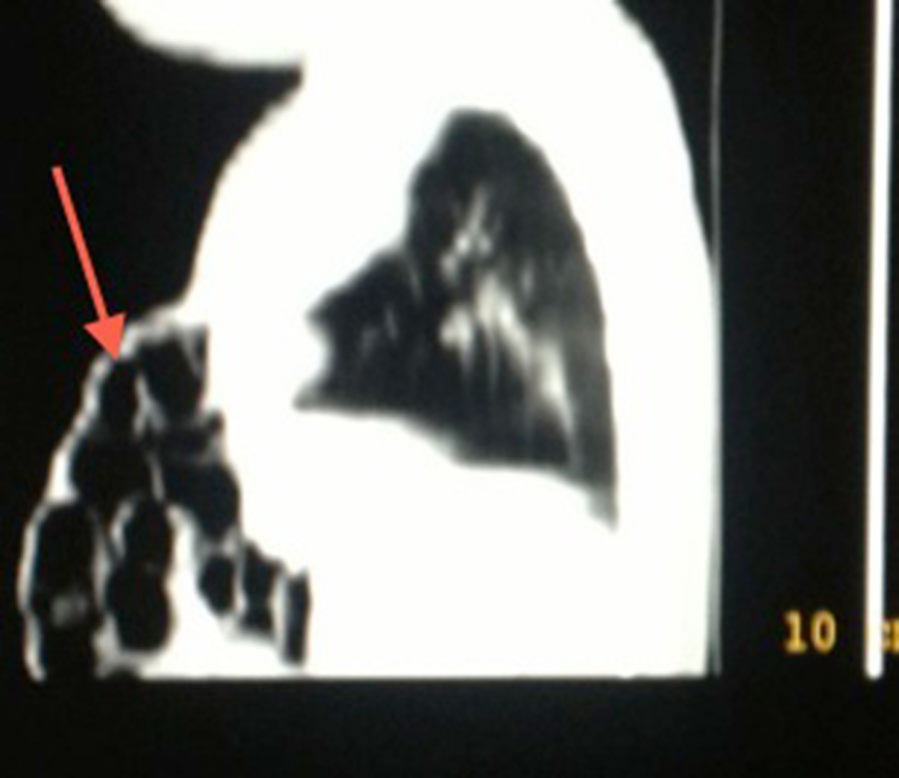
CT scan of the patient done during first month of life, showing loops of bowel protruding in the anterior abdominal wall defect (demonstrated by the *arrow*).
